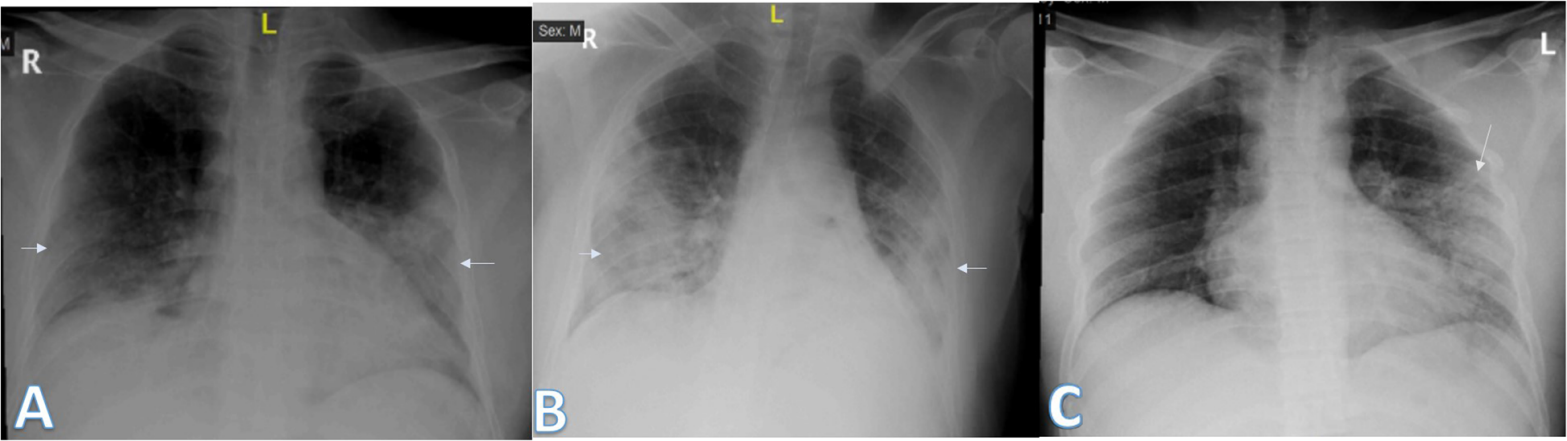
Three different patients with positive COVID-19. a 29-year male was complaining of SOB, fever, and cough. Initial chest X-ray shows bilateral lower zonal air space consolidation opacities (arrows). The severity score was 2 for each lung, so TSS was 4. b 52-year male was complaining of dyspnea and fever. Initial chest X-ray shows bilateral air space consolidation opacities (arrows) at both mid and lower lung zones (with lower zonal predominance). The severity score was 3 for each lung, so TSS was 6. c 32-year male patient was complaining of SOB and cough. Initial chest X-ray shows air space consolidation opacity (arrow) at the left mid and lower lung zones (with lower zonal predominance). The total severity score was 3
